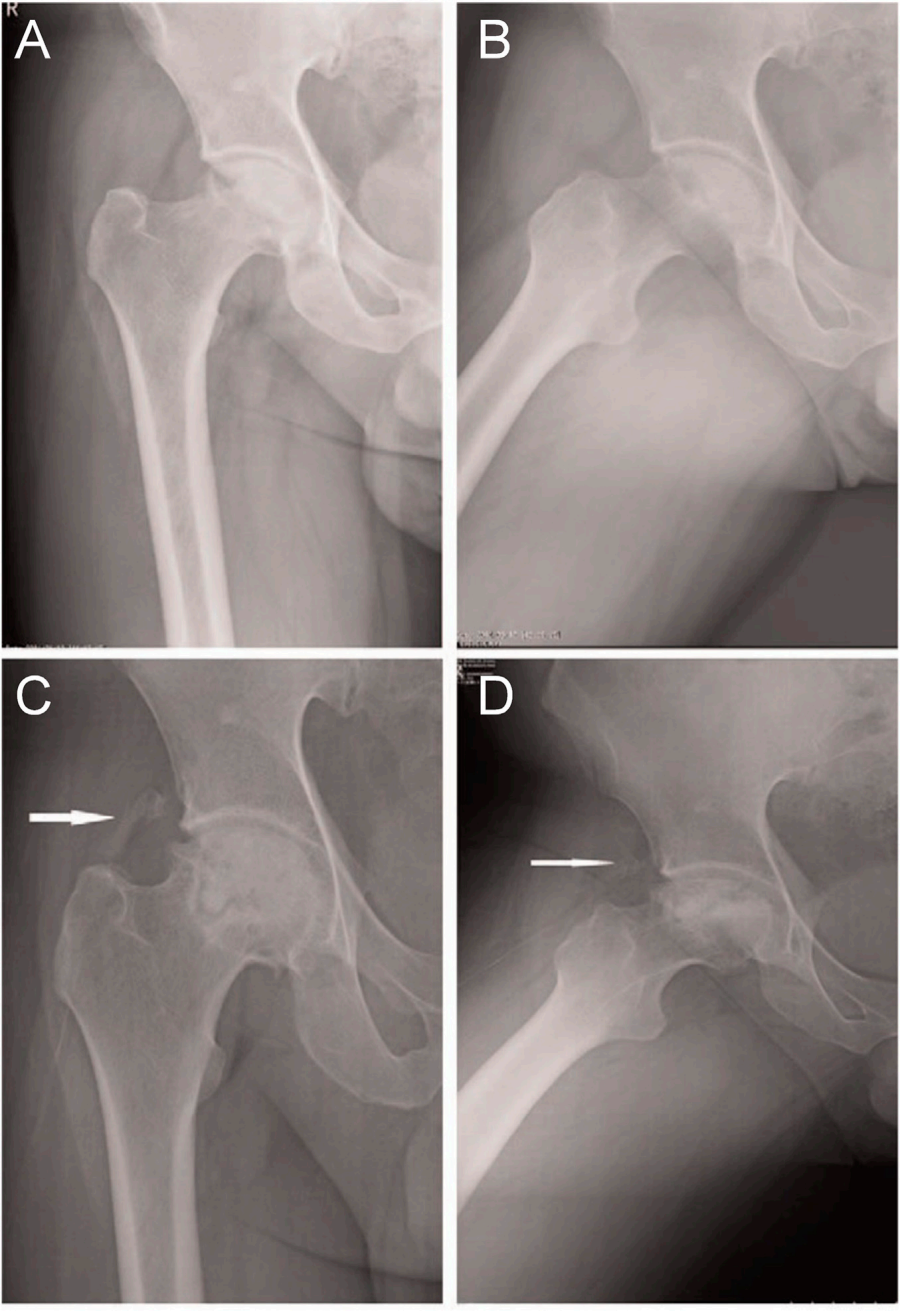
(A): Osteonecrosis of the right femoral head in a 36-year-old male patient with ARCO stage IIIa disease. Graphs (A) and (B) represent the preoperative anteroposterior and frog-leg lateral X-ray images of the right hip, respectively. Graphs (C) and (D) represent the postoperative anteroposterior and frog-leg lateral X-ray images, respectively, after 4 months of surgery, showing a lamellar fragment of HO (class II) in the anterolateral soft tissues of the hip joint (white arrow). ARCO, Association Research Circulation Osseous; HO, heterotopic ossification. Reprinted with permission from Shi et al. (2017).
